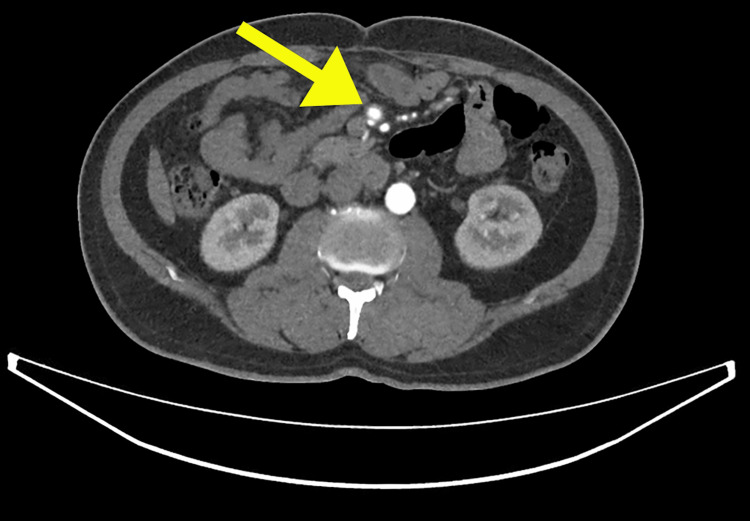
Axial CT aortography demonstrating superior mesenteric artery dissection. Partial thrombosis of the false lumen is seen (arrow), with moderate narrowing of the true lumen and preserved distal contrast opacification. CT, computed tomography
